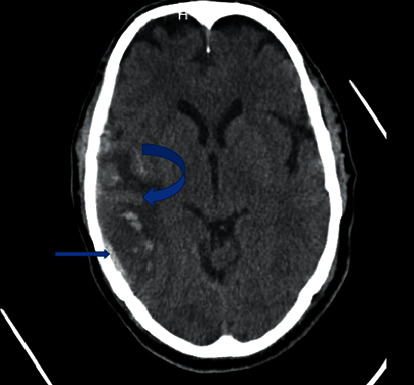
First brain CT scan showing cortical vein thrombosis (inferior anastomosis vein) (rectus arrow) with right temporal infarction in which transformation to hemorrhage occurs (curved arrow).
